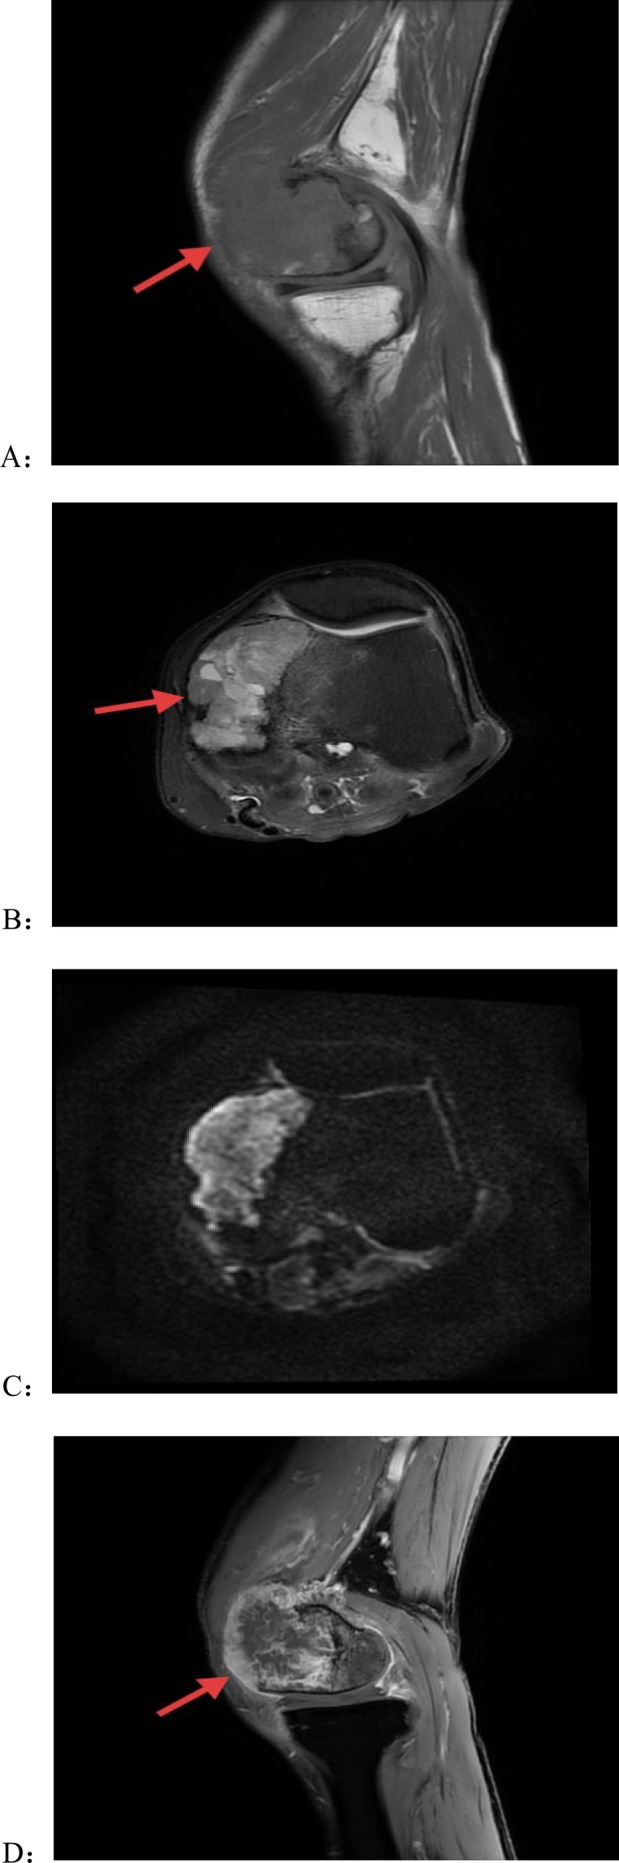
Preoperative contrast‐enhanced magnetic resonance imaging (MRI) images of the left knee joint: Expansile bony destruction is seen in the left femoral medial condyle, with mass‐like isointense T1 (A) and heterogeneous hyperintense T2 (B) signals, measuring approximately 57 × 26 × 50 mm with clear boundaries. The lesion exhibits heterogeneous hyperintensity on diffusion‐weighted imaging (DWI) and heterogeneous enhancement on contrast‐enhanced scan (C). Patchy non‐enhancing T2 hyperintensities are noted within the lesion. Patchy T1 hypointense and T2 hyperintense signals are present in the adjacent bone (D).
